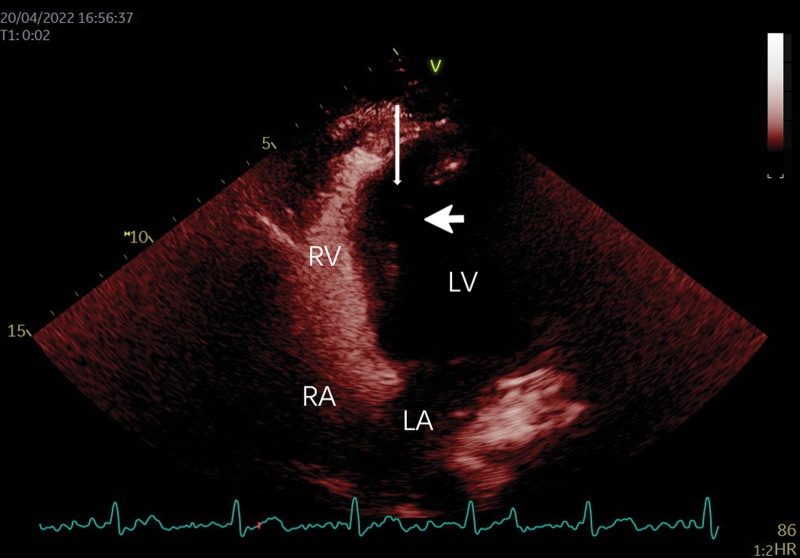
Contrast enhance-ultrasound (CEUS) imaging. The apical 4-chamber view showed the right atrium and right ventricle were sequentially visualized, no contrast was visualized in the bilayer structure of the ventricular septum (long arrows) or in the left ventricular cavity and the continuity interruption (short arrows). There were no negative contrast areas in the right ventricular cavity.
